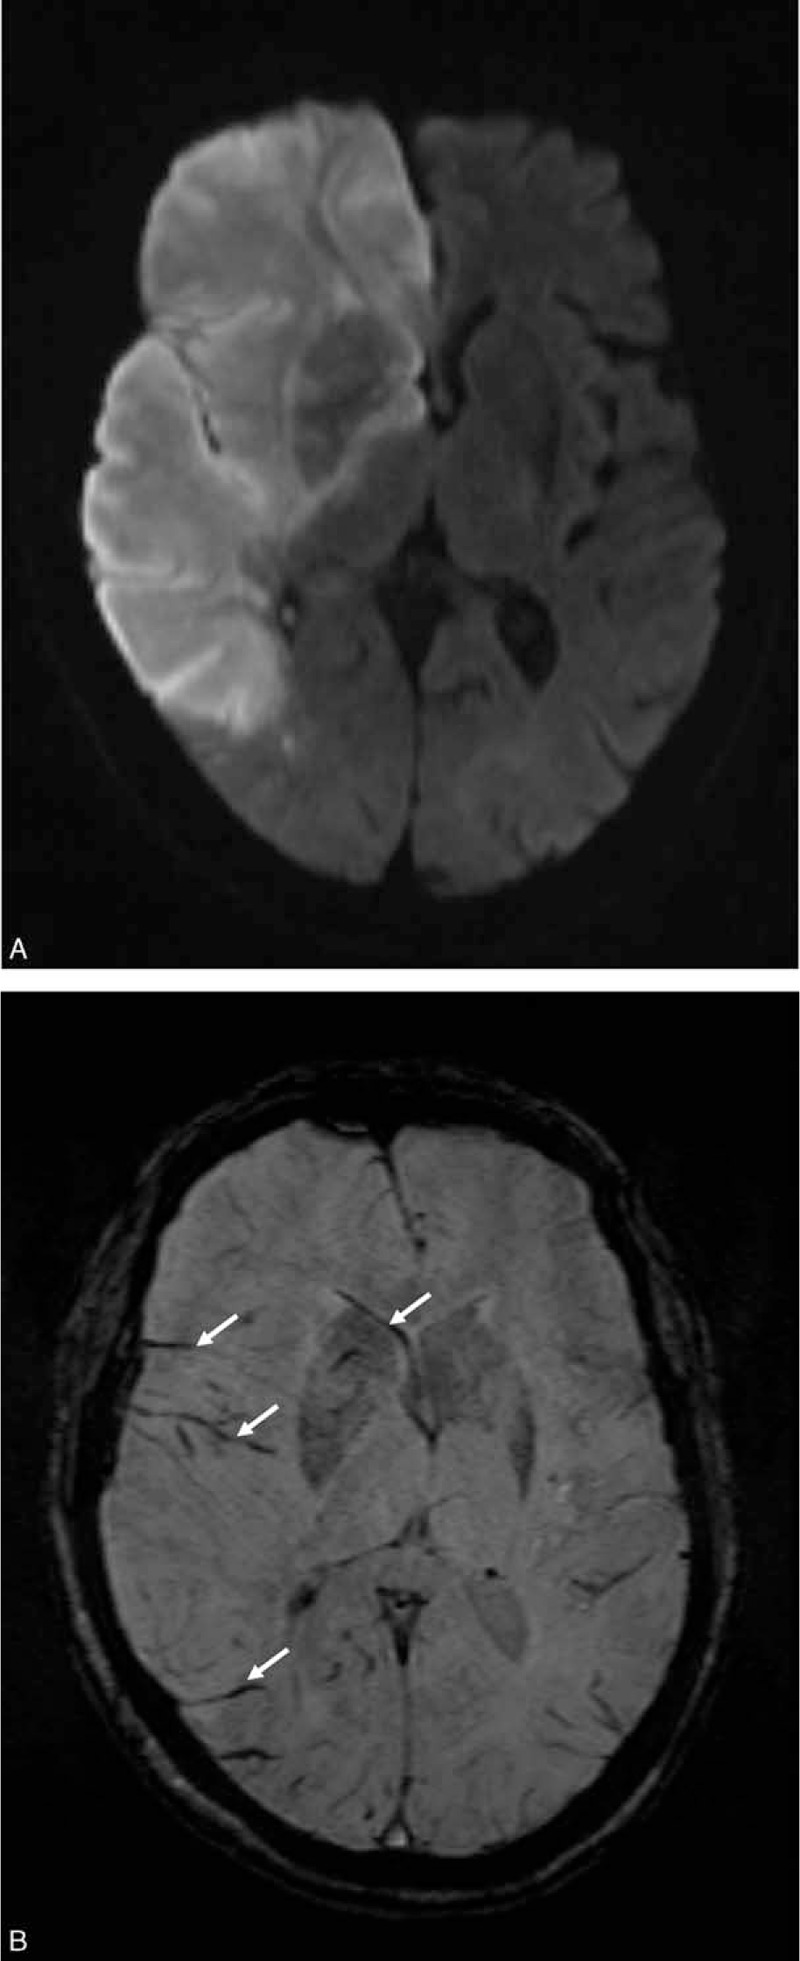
In a 79-year-old woman who died from acute right middle cerebral artery infarction, MRI was performed 2 days after symptom onset. (A) Diffusion-weighted images show hyperintensity in the right cerebral hemisphere including the frontal, parietal, and temporal lobes; insula; basal ganglia; and internal and external capsules. The Stroke Program Early MR Score (SPEMRS) was 0. (B) On susceptibility-weighted imaging, the SPEMRS value was 0 as asymmetric prominent vessels (arrows) were in the insula, internal capsule, caudate nucleus, lentiform nucleus, and all areas of the middle cerebral artery territory.
